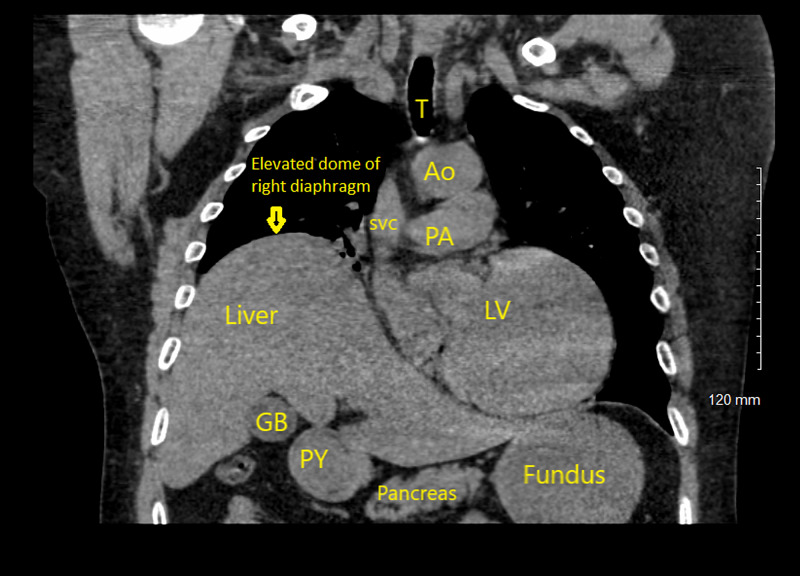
Coronal section of non-contrast CT Chest depicting the elevated dome of right hemidiaphragm with adjacent structures of gall bladder (GB) , pylorus of stomach (PY), left ventricle (LV), trachea (T), aorta (Ao), pulmonary artery (PA) and superior vena cava (SVC).
